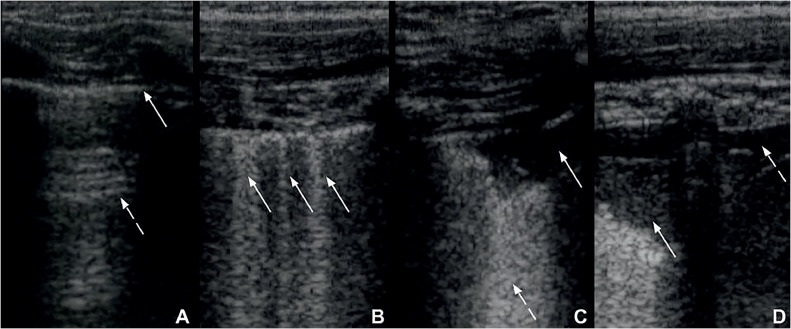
A. Lung ultrasound obtained with linear probe showing continuous and regular pleural line (continuous arrow) and presence of A-lines (dashed arrow), horizontal hyperechogenic artifacts due to the high reflectivity of the normally aerated lung. B: Lung ultrasound obtained with linear probe, showing presence of B-lines (continuous arrows), vertical white lines, due to alterations in the acoustical properties of the lung, representing the partial replacement of air in the alveolar space by fluid. C: Lung ultrasound obtained with linear probe, showing a small consolidation (continuous arrow), associated with hyperechogenic areas below (dashed arrow), representing the loss of aeration of lung parenchyma. D: Lung ultrasound obtained with linear probe, showing a larger consolidation (continuous arrow) and hyperechogenic area below, associated with a small pleural effusion (dashed arrow).•One point: the pleural line is indented. Below the indent, vertical white lines (B-lines), due to local alterations in the acoustical properties of the lung are visible, representing the partial replacement of the air in the alveolar space by fluid (Figure 2B).•Two points: the pleural line is broken. Below the breaking point, consolidated areas (hypoechogenic areas-subpleural consolidations) appear with associated hyperechogenic areas below the consolidated area (white lung – coalescent B-lines). This represents the loss of aeration and the transition of these areas toward acoustic properties like soft tissue over the entire area represented by the consolidation itself. Pleural effusion might or might not be identified (Figure 2C).•Three points: the scanned area shows dense and largely extended white lung with or without larger consolidations. Pleural effusion might or might not be identified (Figure 2D).
